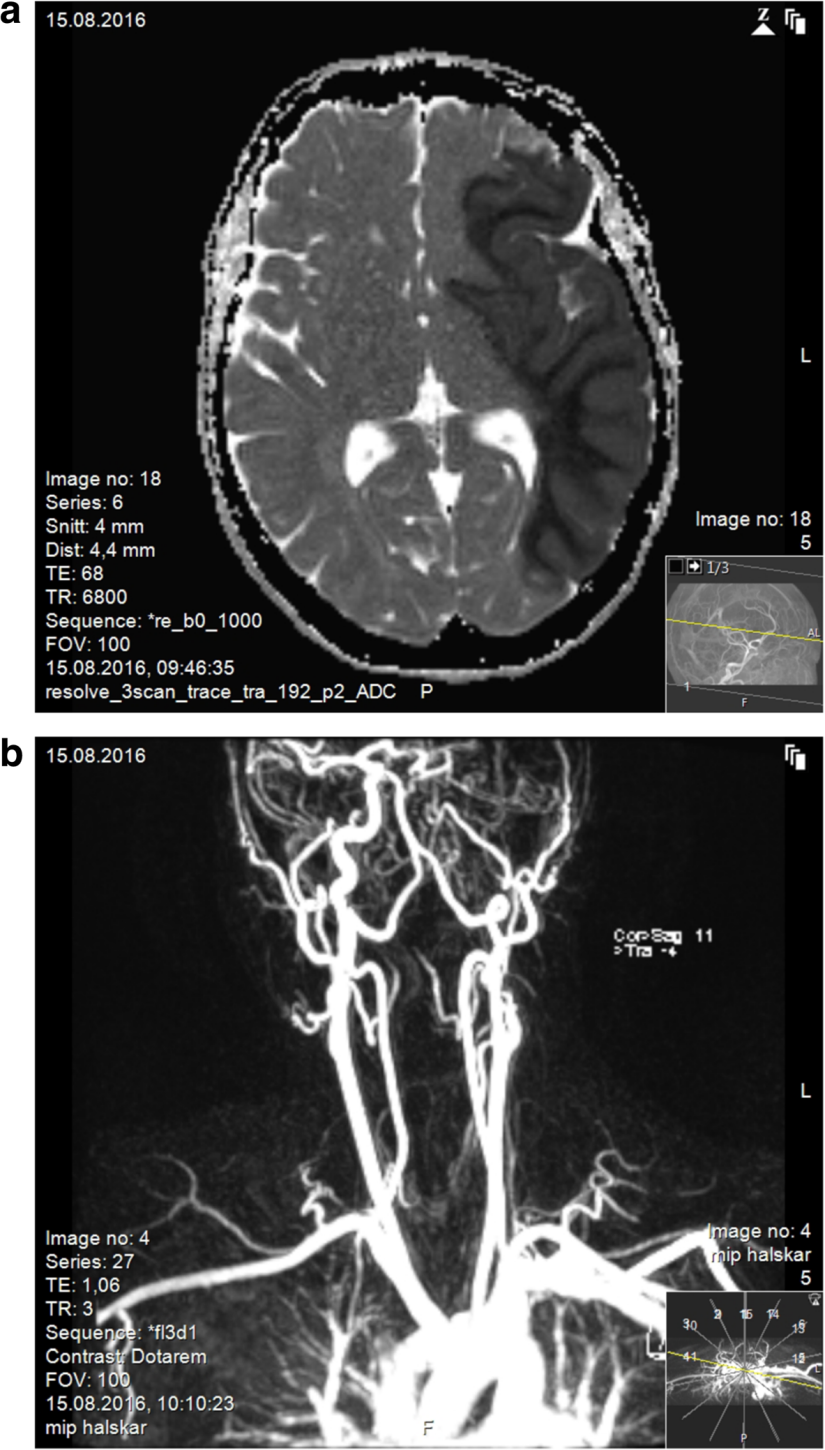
a Head magnetic resonance imaging taken 17 hours after admittance (*top*); Shows an extensive left middle cerebral artery infarction. b Magnetic resonance angiography taken 17 hours after admittance (*bottom*); Shows an occluded internal carotid artery on the left side approximately 1 cm above the bifurcation, in addition there is occlusion of the left middle cerebral artery
